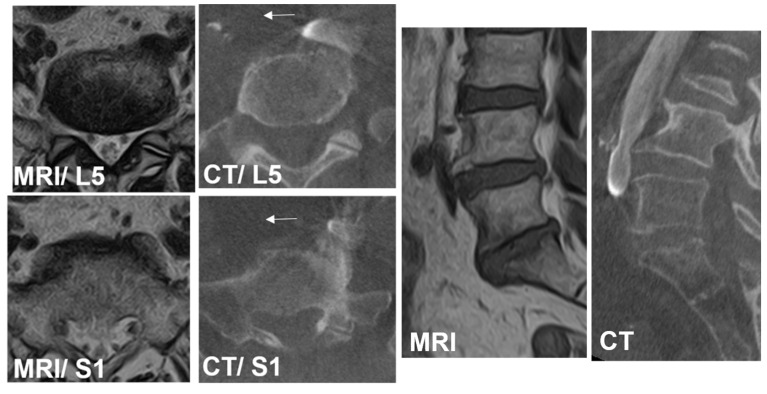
A 75-year-old woman with L4 degenerative spondylolisthesis and L5/S1 degenerative disc disease received L4-S1 OLIF followed by lateral position percutaneous screwing and fixation. The comparison between preoperative supine MRI images and intraoperative vein-enhanced CT demonstrated left CIV movement of 6.7 mm to the right in the right decubitus position at the L5 lower endplate level, and 3.4 mm to the right at the S1 upper endplate level (arrows). The vein bifurcation moved 8.5 mm to the caudal direction under the right decubitus position.
